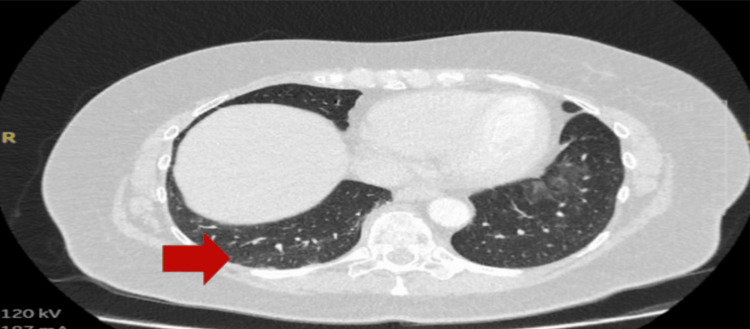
High-resolution CT of the chest showing subtle right middle lobe ground-glass changes (red arrow).
